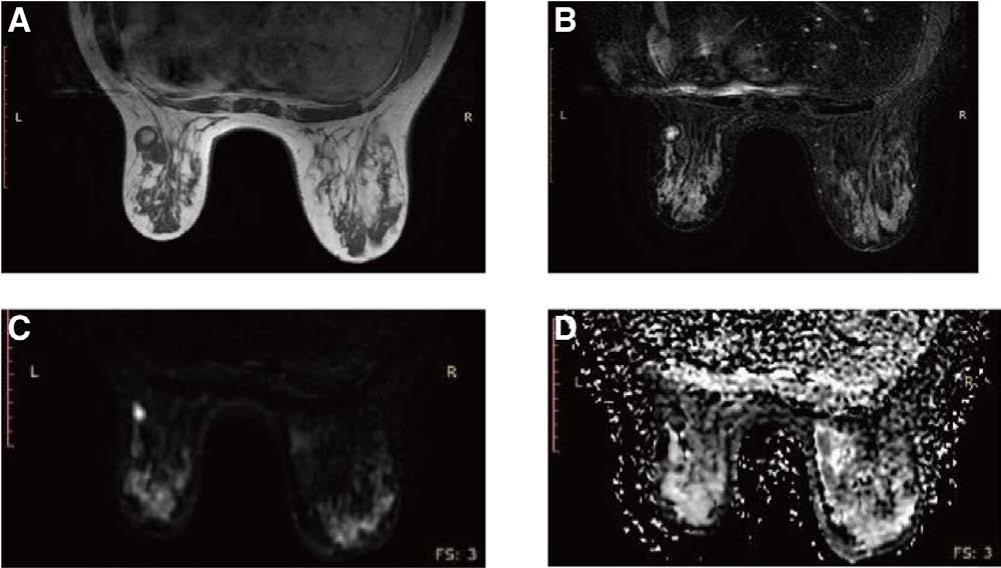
(A) T1-weighted fat saturated (T1WFS) sequence magnetic resonance imaging (MRI) of a mass in the outer lower quadrant of the left breast demonstrated a tumor with uniformly high internal signal intensity. (B) T2-weighted short-time inversion recovery (STIR) MRI demonstrated an uneven signal intensity tumor with low signal intensity of the external edge. (C, D) The tumor showed high signal intensity on diffusion weighted image (DWI) (C) and a signal cavity on the apparent diffusion coefficient (ADC) graph (D).
